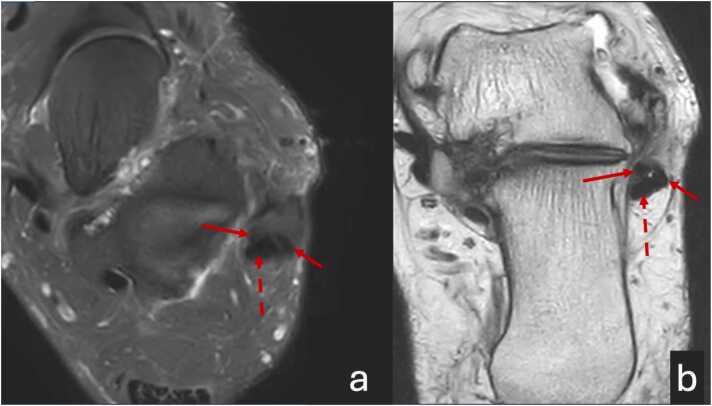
A 52-year-old patient with pain behind the lateral malleolus for approximately 12 months. The MRI scan: a - transverse proton density with fat suppression, b - T2-weighted image. The MRI shows a complete split rupture of the peroneus brevis (solid red arrow). The peroneus longus tendon is normal and is marked with a dashed arrow.
